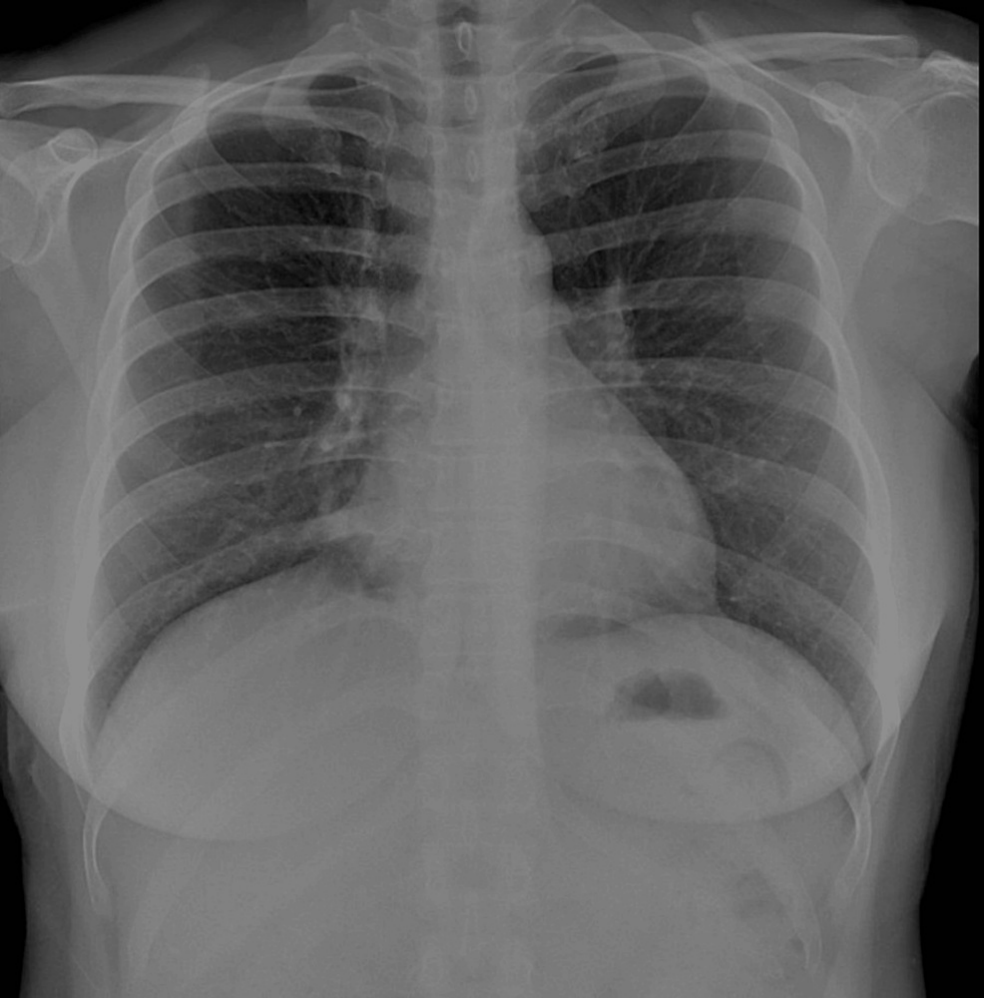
Postoperative chest X-ray confirming successful recovery
